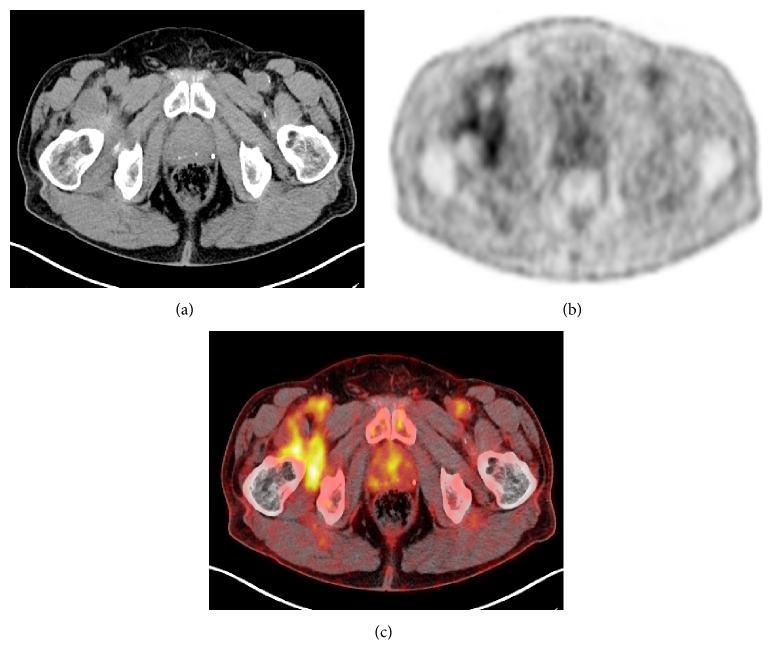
(a) Computerized tomography (CT) axial scan. (b) Fluorine-18-fluorodeoxyglucose positron emission tomography (^18^F-FDG PET) axial scan. (c) ^18^F-FDG-PET/CT fusion image. A moderate uptake in the anterior-lower aspect of the right hip joint is appreciable, more evident in the peripheral portion of the neoformation. A remarkable uptake is present in right hip joint.
